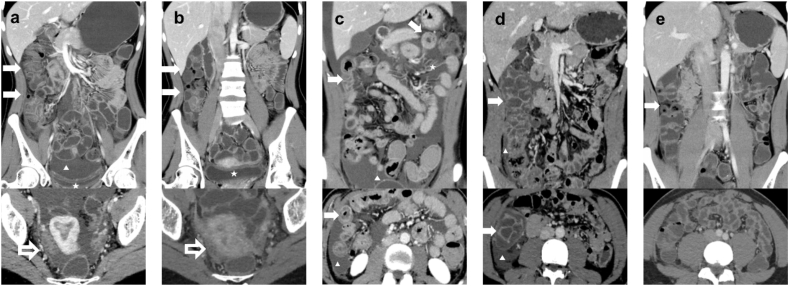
Abdominal CT images of gastrointestinal edema onset and remission in 2 patients. (a-b) This is a 26-year-old female patient who presented with a one-day history of abdominal pain and subsequently underwent both non-contrast and contrast-enhanced CT imaging. The upper image of fig. 5a demonstrates edema and thickening of the right colon along (→) with pelvic effusion (▲), as well as edema of the bladder wall (★). The lower image of fig. 5a reveals mild ovarian swelling (→). Following admission, the patient's abdominal pain resolved after receiving icatibant medication. A follow-up CT (Fig. 5b) examination conducted the next day indicated that the thickening of the right colon has largely subsided, ascites is fully absorbed, there is significant improvement in bladder wall edema (★, upper image of fig. 5b); and the lower image of fig. 5b confirmed resolution of bilateral ovarian swelling (→). (c-e) A 21-year-old male patient presented to our hospital for the first time with complaints of abdominal pain. Initial coronal and axial CT imaging (Fig. 5c) revealed edema and thickening of the small intestine in the mid-to-upper abdomen (→), characterized by target sign, along with surrounding ascites (▲) and comb sign features (★). One month later, the patient was readmitted due to recurrent abdominal pain. CT imaging (Fig. 5d) demonstrated significant edema and thickening of the right colon (→), with more pronounced target sign, and a small amount of ascites (▲) was noted. Upon admission, icatibant therapy was initiated. A subsequent CT conducted 1.5 days later (Fig. 5e) indicated complete resolution of all previous abnormal imaging findings, coinciding with the alleviation of abdominal pain symptoms.
